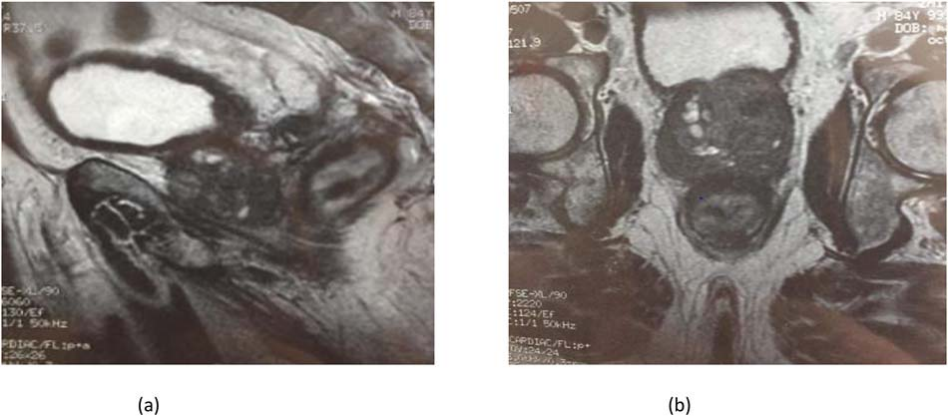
(a) MRI features: circumferential wall thickening narrowing the rectal lumen and invading the prostate and the mesorectum without extrinsic mass on sagittal section of the pelvis. (b) MRI features: a cross section of the pelvis showing circumferential wall thickening narrowing the rectal lumen and invading the prostate and the mesorectum.
